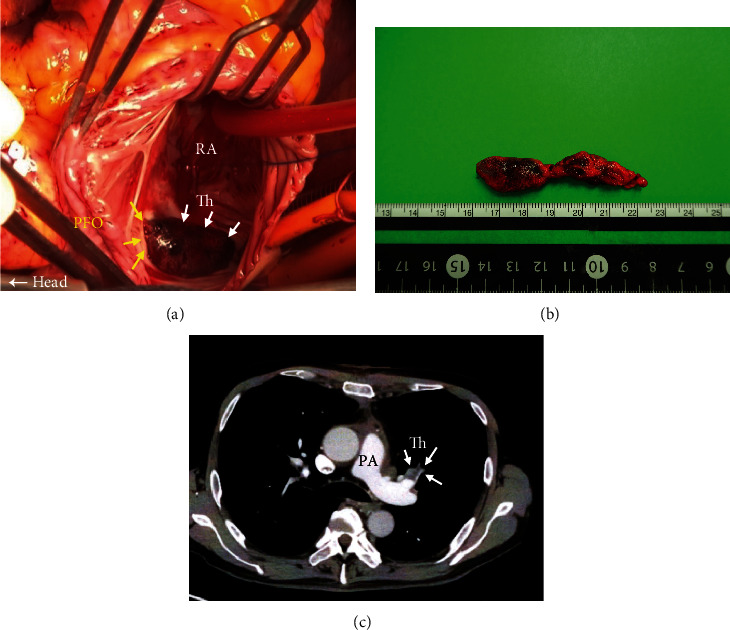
Intraoperative picture, resected thrombus, and postoperative CT findings. Intraoperative picture showed an entrapped large thrombus in the PFO (a) and resected large thrombus (b). Yellow arrows indicated the PFO, and white arrows indicated an entrapped thrombus, respectively (a). Postoperative CT showed the disappearance of the thrombus in the left main PA (c). White arrows indicated the residual thrombus in the left PA. PFO: patent foramen ovale, CT: computed tomography, PA: pulmonary artery, Th: thrombus.
